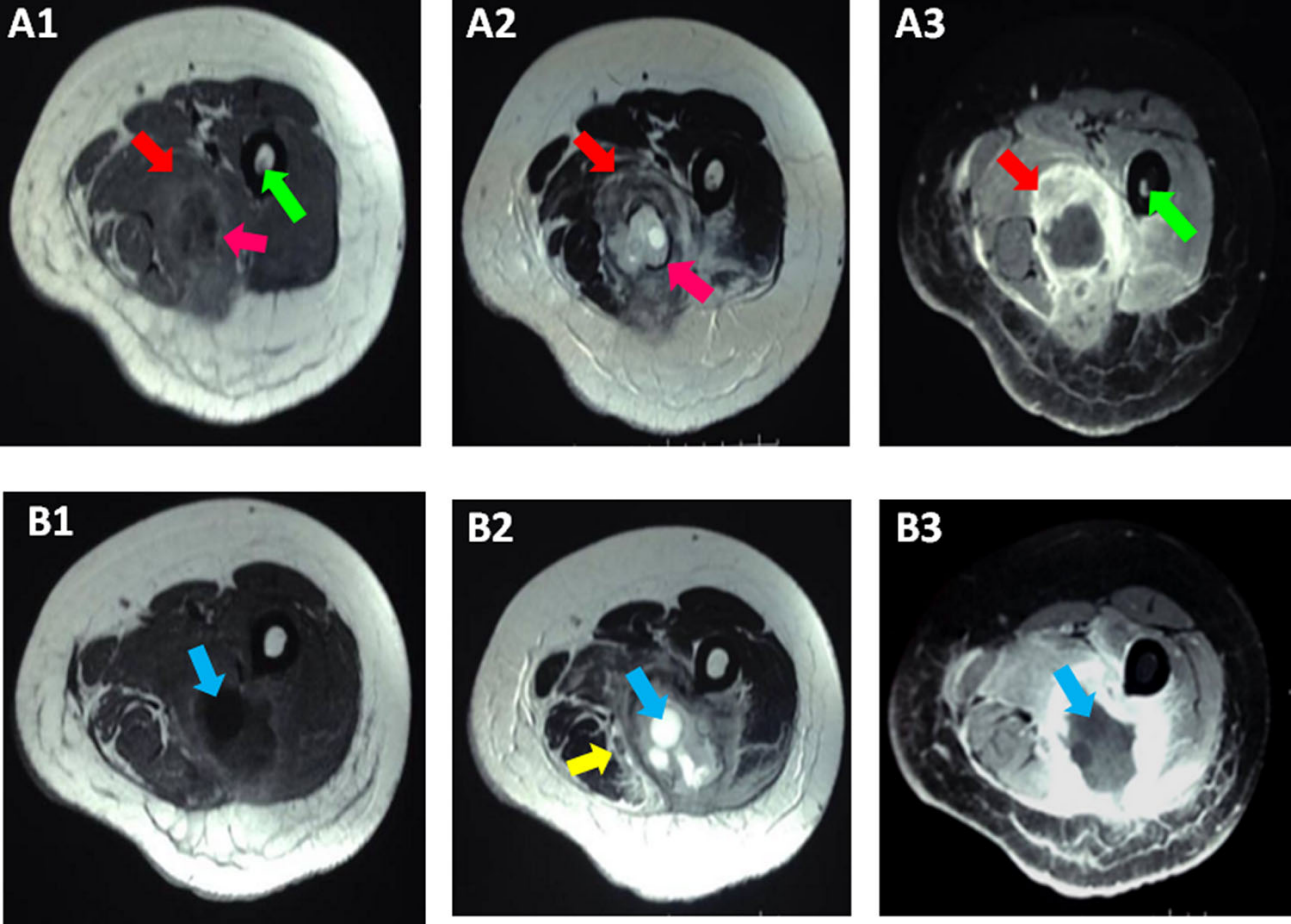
MRI of the left thigh in two different cutting levels (A1+A2+A3) and (B1+B2+B3). Axial TSE T1-weighted images (A1+B1), TSE T2-weighted images (A2+B2) and contrast-enhanced fat-suppressed TSE T1-weighted images demonstrated an intramuscular cystic mass of the middle one-third of the posterior compartment of the left thigh within the biceps femoris muscle. This mass is delimited by a discontinuous rim of low T2 and high T1 signal "rim sign"(pink arrow) and contains multiple well defined cystic lesions of more intense high T2 and low T1 signal corresponding to daughter cysts (blue arrow) with a "cyst within a cyst” appearance. This cystic mass is surrounded by an edematous infiltration of the adjacent muscles with low T1 and high T2 signal and avid enhancement after contrast administration (red arrows). Enhancement of the muscular fascia and of the subcutaneous fat of the posterior aspect of thigh. The mass represses the sciatica nerve without invading it (yellow arrow) and is distant from the profound and superficial femoral pedicles. A low T1 signal of the spongiest bone enhanced after contrast administration was noted (green arrow).
